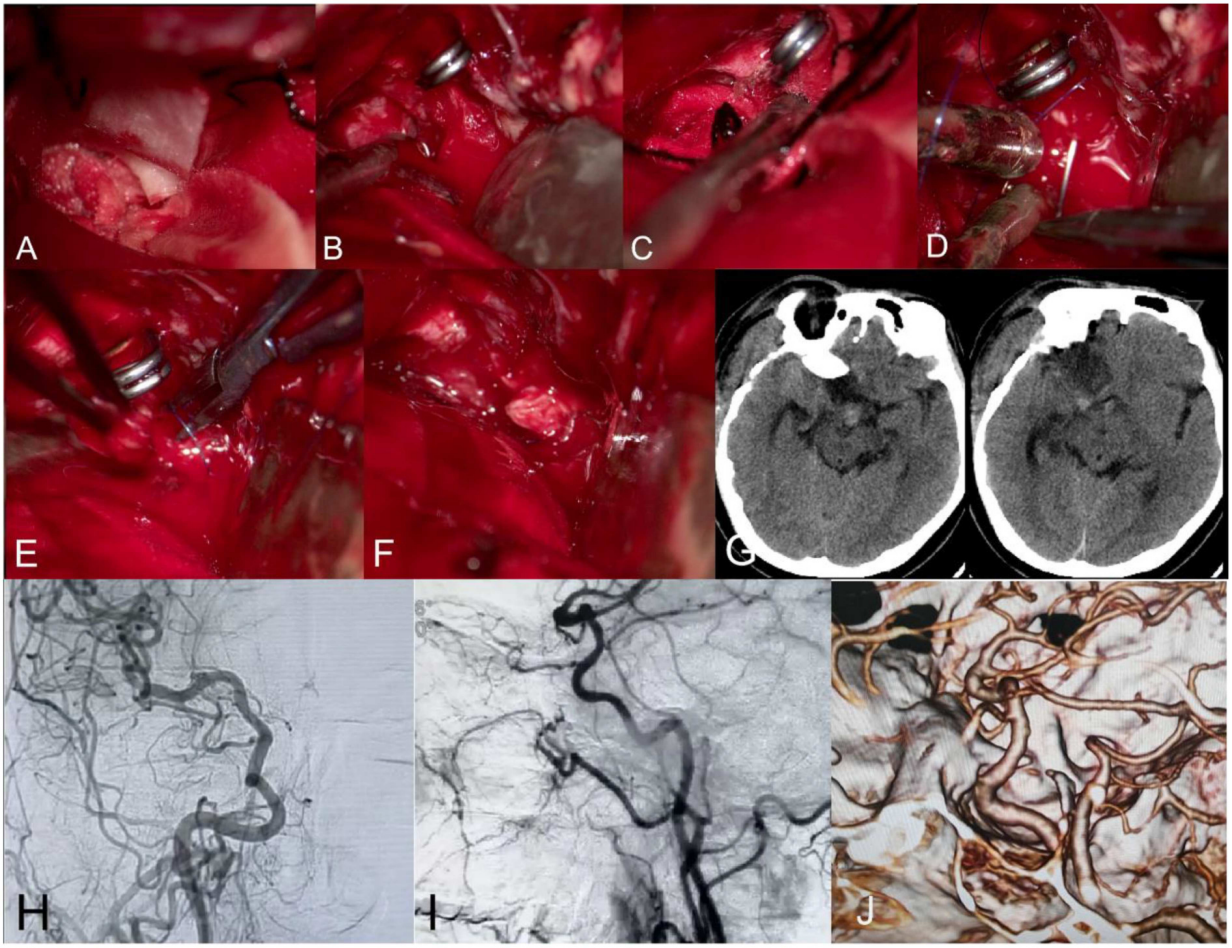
(A) compression hemostasis by hemostatic sponge and cotton pads; (B) separate and block the C6 segment of the internal carotid artery temporarily; (C) expose the rupture of the internal carotid artery; (D,E) muscle tissue is covered by the rupture of the internal carotid artery, and the rupture is repaired by micro-suturing; (F) temporary blockage removed and hemostasis achieved successfully; (G–I) postoperative CT and DSA. (J) 6-months follow-up CTA.
